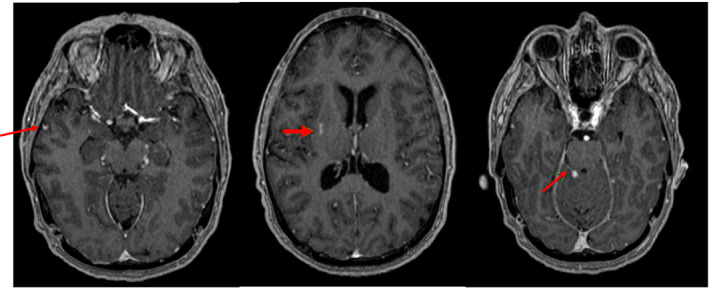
MRI brain with contrast, post‐contrast T1 weight sequences. There are multifocal T2/FLAIR hyperintensities in the right superior cerebellum, left thalamus, bilateral temporal lobes, and right basal ganglia, all associated with mild parenchymal or leptomeningeal enhancement.
